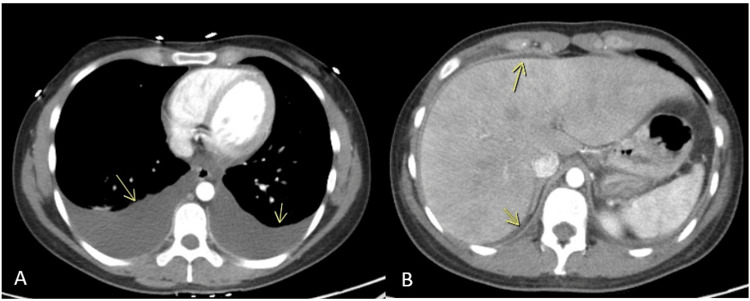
A computerized tomography of the abdomen and pelvis demonstrating interval increase in ascites and pleural effusions. (A) Sagittal views of a CT demonstrating moderate bilateral pleural effusions, right more than left, significantly increased from the prior examination. The pleural effusions are indicated by the arrows. (B) Moderate volume ascites noted in the abdomen and pelvis increased in volume since the prior exam, as indicated by the yellow arrows.
